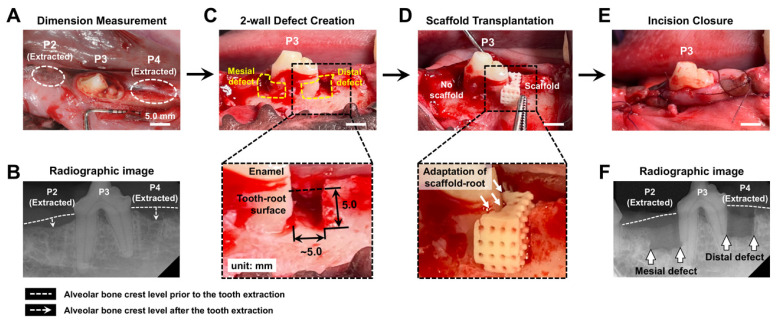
Surgical creation of the 2-wall periodontal defect and scaffold transplantation after tooth extraction socket healing. (A,B) After extracting the 2nd and 4th premolar teeth (P2 and P4), the X-ray radiograph showed natural bone healing within 12 weeks. (C) Periodontal 2-wall defects were surgically created around the mesial and distal roots of the 3rd premolar tooth (P3) with specific dimensions, and curettes were utilized to remove the cementum and periodontal ligaments on the tooth-root surface. (D) One defect received the perio-complex scaffold transplantation with the geometric adaptation between the scaffold and tooth-root, while the other was left vacant. (E,F) After the flap was sutured in position, X-ray radiography provided the experimental defect sites around the P3 tooth. White arrows indicate horizontal defect margins, and white dashed lines represent the borderline of the original bone crest level. Scale bar, 5.0 mm.
